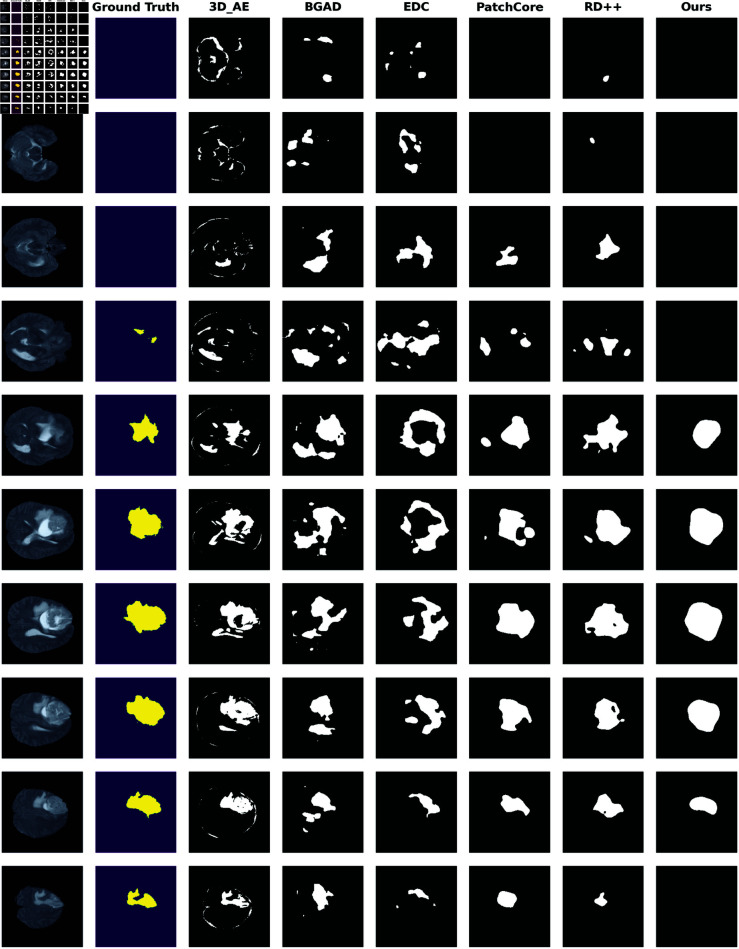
Qualitative Results on the SOTA methods on a single T2 volume. This figure displays intermediate slices in sequence from a single volume selected from the testing dataset. The visualization is designed to demonstrate how well the model performs spatially across an entire volume. The first row presents the images (slices) from brain MRI volumes and the second row shows the ground-truth for the anomalous regions. The remaining rows depict the binary anomaly maps predicted by SOTA models. These maps are generated by applying the F1 threshold, which achieves the best F1 score, to convert anomaly scores into binary masks.
